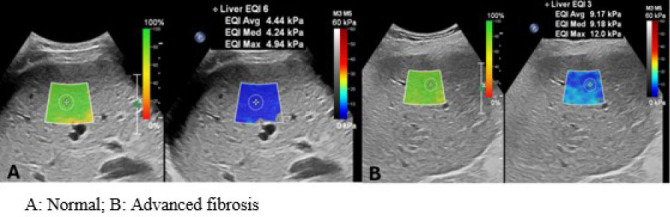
Example of ultrasound-based liver elastography using the 2D-SWE
